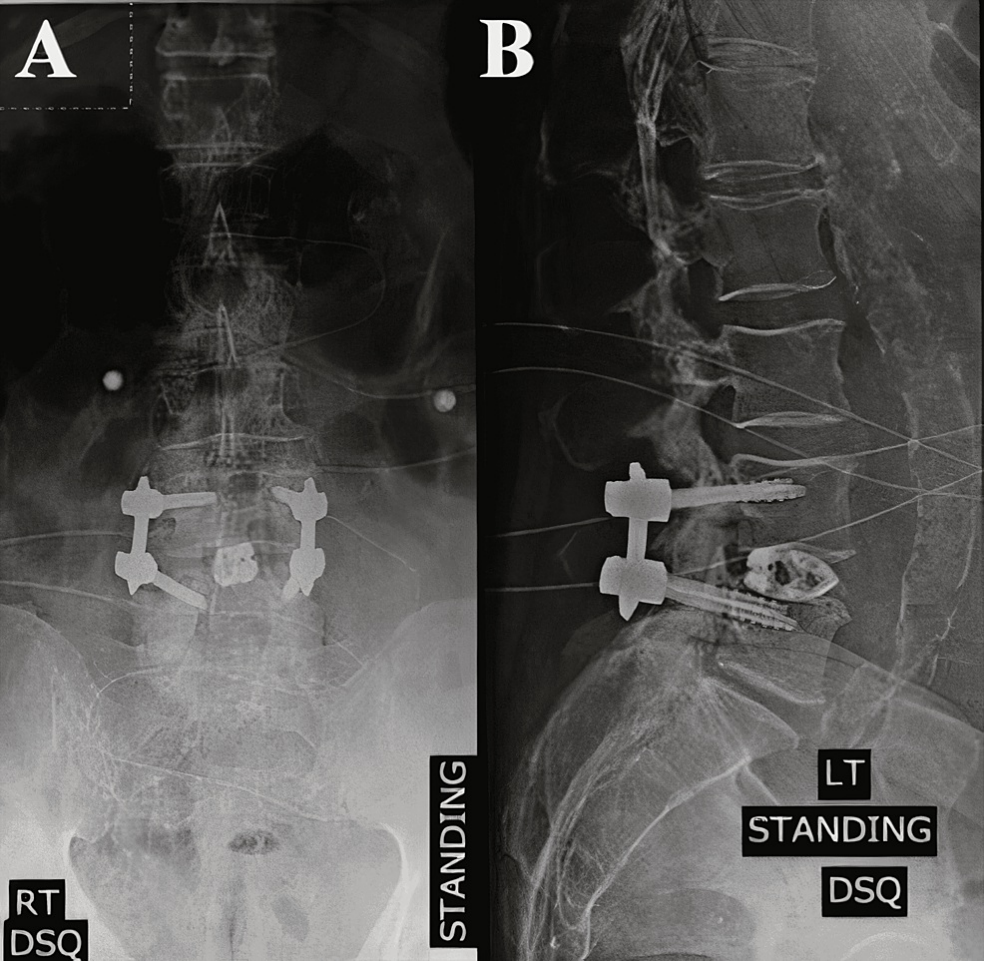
AP (A) and lateral (B) postoperative lumbar spine X-rays
